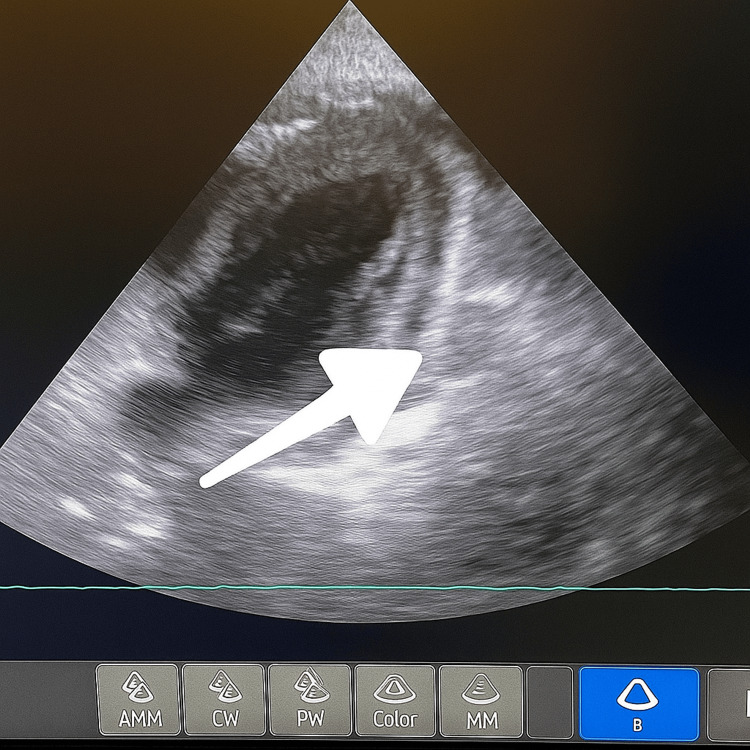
Bedside echocardiogram. The arrow indicates pericardial effusion.
